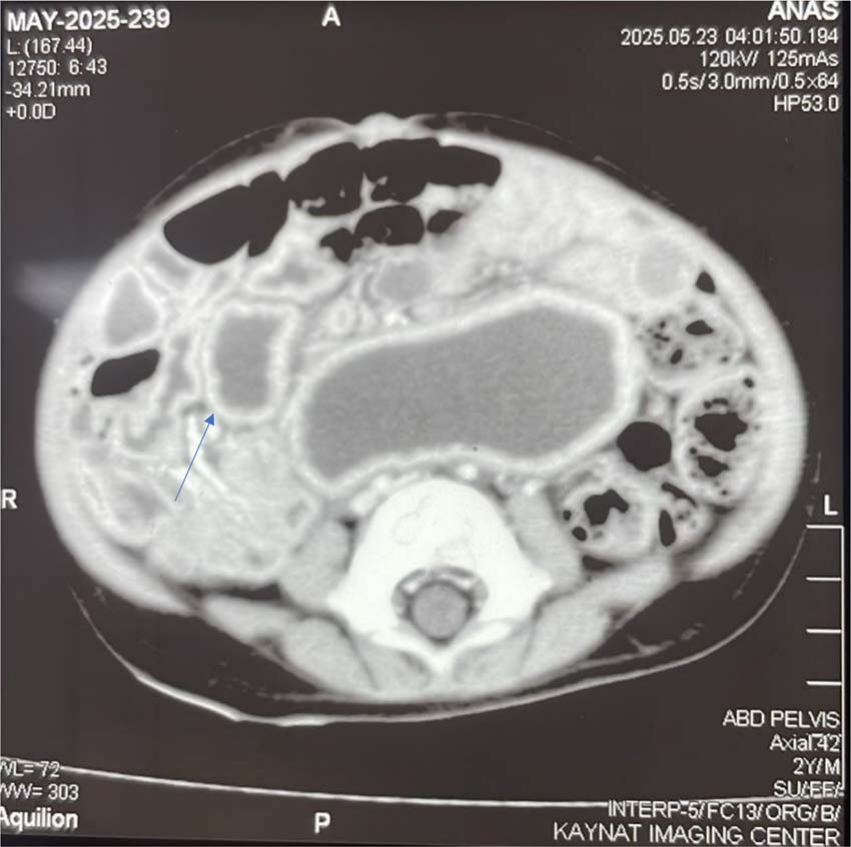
Contrast-enhanced axial CT scan of the abdomen. The image reveals a well-defined, thick-walled cystic lesion (arrow) located along the mesenteric border of the midgut. The lesion demonstrates peripheral post-contrast enhancement without internal septations or solid components, findings highly suggestive of a jejunal duplication cyst.
